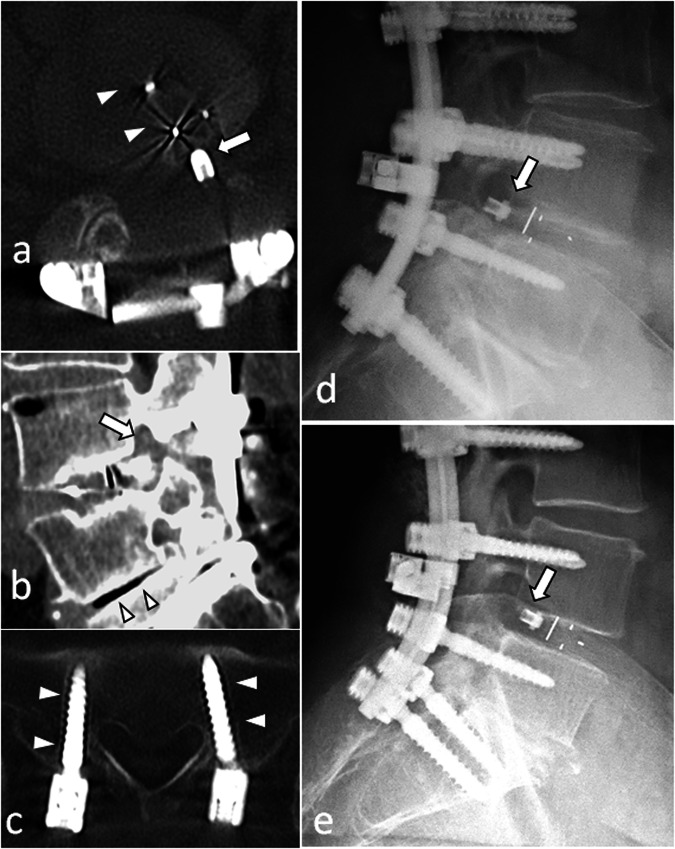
Patient referred for a left cruralgia that occurred after a 2 years pain-free interval following a lumbar arthrodesis with transforaminal lumbar interbody fusion (TLIF) at L4–L5 level. On axial CT-images with har kernel reconstructions (a) the interbody spacer (arrowheads), is lateralised, which is expected after TLIF surgery, but its posterior parts is seen beyond the disk limits (arrow). Sagittal soft kernel reconstruction (b) shows a narrowed right L4–L5 foramen, the spacer occupying the inferior part of the foramen. Conspicuous analysis reveals thin bone resorption around the S1 screws (arrowheads) (c). On contemporary lateral radiographs (d), the spacer seems too posterior. Comparison with baseline radiographs (e) confirms a posterior displacement of the spacer, which is diagnostic for pseudarthrodesis
